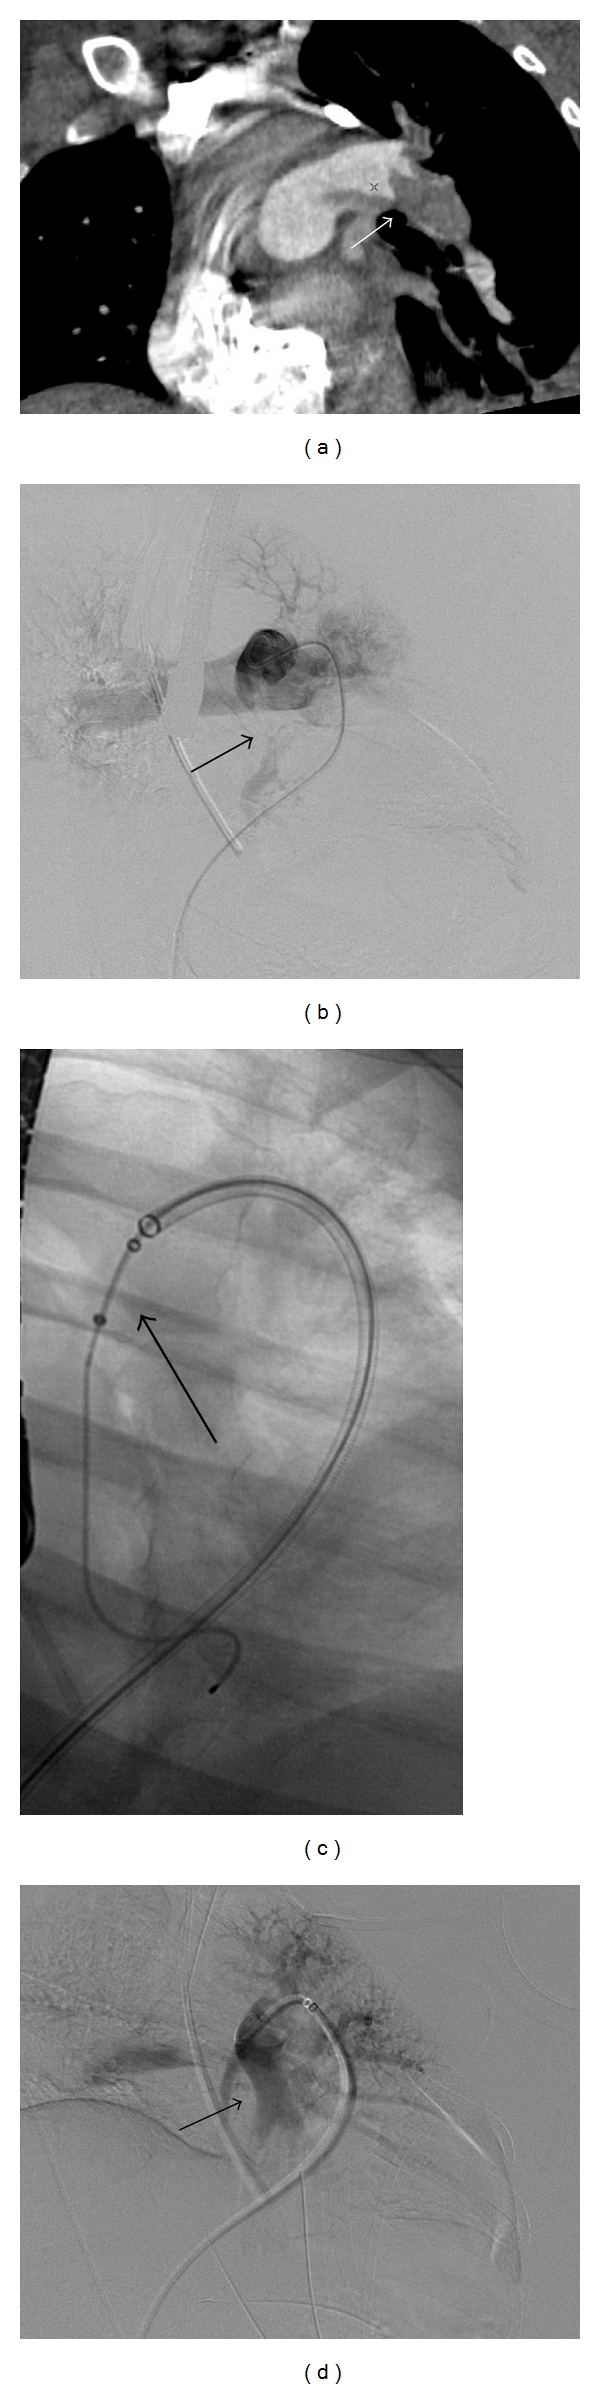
This is 44-yr-old woman with acute chest pain shortness of breath and tachycardia. Oblique coronal CT image (a) and pulmonary angiogram (b) showed massive left main and lower lobe PA embolus (arrows). Mean pulmonary artery pressure on initial study was 34 mm Hg. A 6-French Angiojet device (arrow) was used (c) followed by catheter-directed thrombolysis for 13 hours. Follow-up angiogram demonstrated significant decrease in clot burden (arrow) (d). Mean pulmonary artery pressure decreased from 34 to 18 mmHg.
